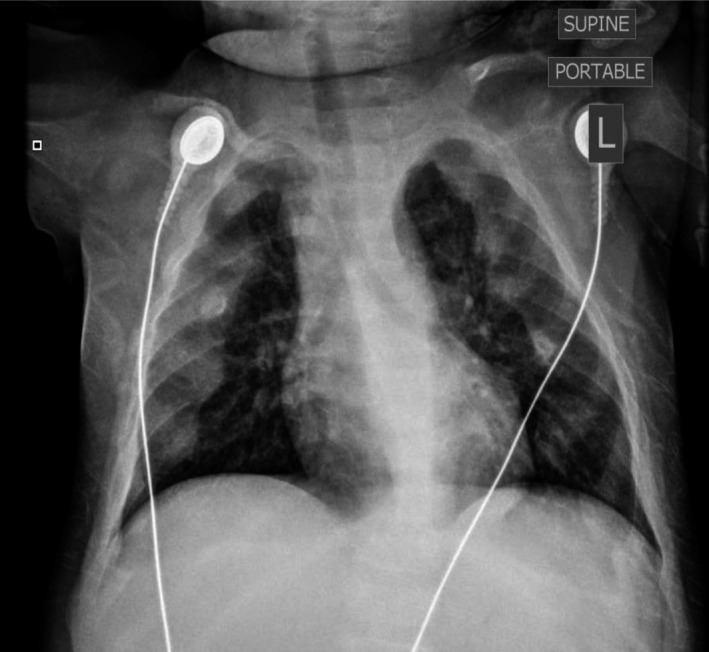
Chest radiograph at admission shows enlarged and confluent appearance of the anterior ribs at the costochondral joints bilaterally consistent with rachitic rosary.
